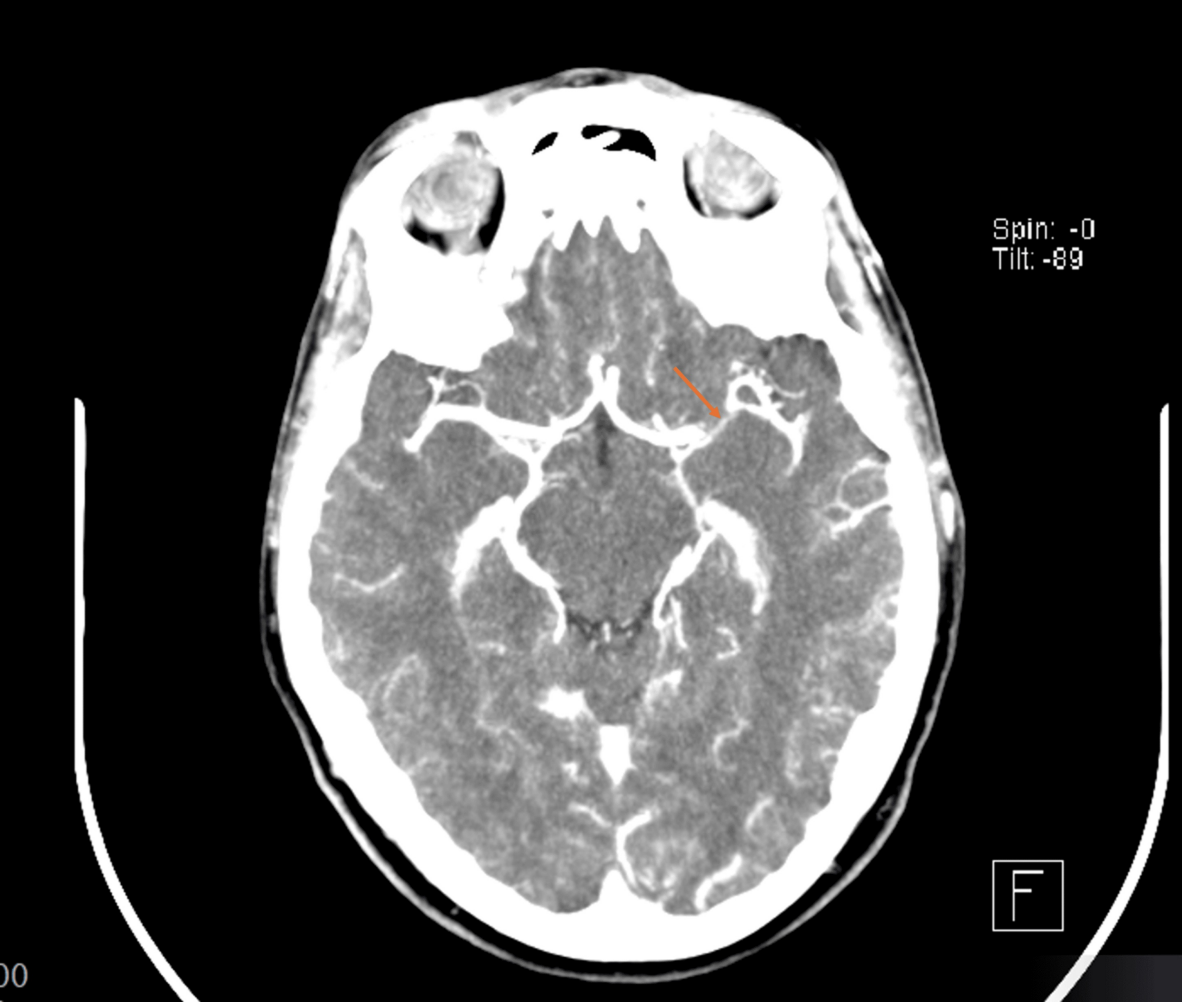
Intracranial CT angiography at initial admission CT demonstrating occlusion of the M1 segment of the left middle cerebral artery (arrow).
